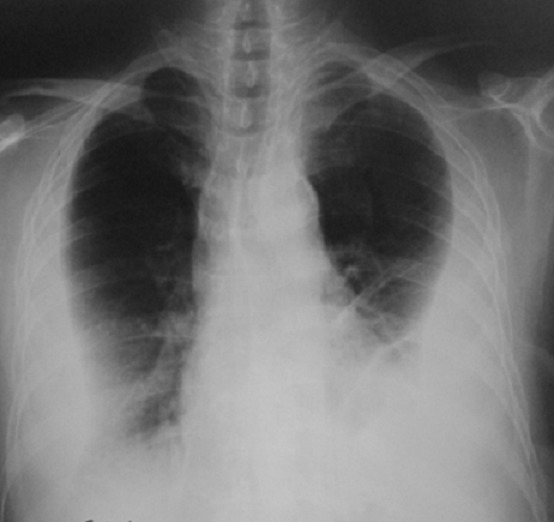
Radiographie du thorax: opacité bilatérale de type pleural
